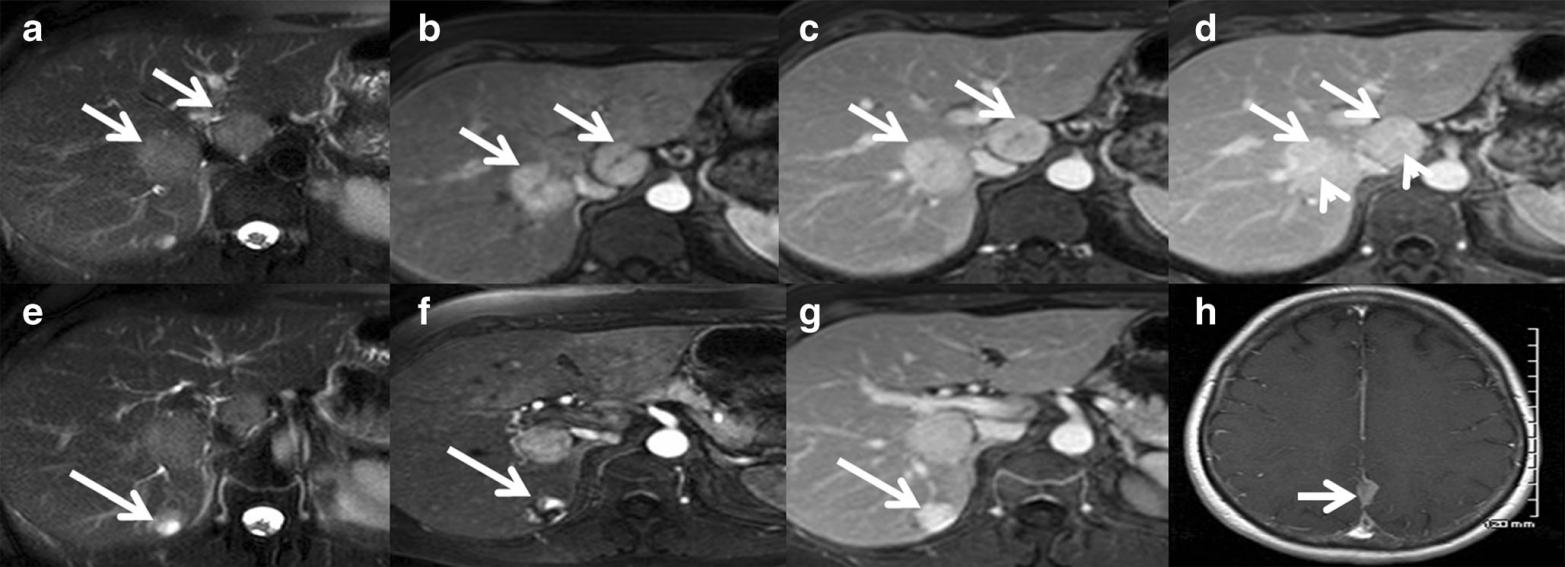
Multiple FNH syndrome in a 50-year-old female. (a) Axial T2W MRI shows two iso- to mildly hyperintense focal lesions (*arrows*) in segments 8 and 4 of the liver. Axial post-contrast MRI shows intense enhancement of the lesions (*arrow*) in the arterial phase (b) and persistent enhancement (*arrow*) in the portal venous (c) and delayed phases (d) with delayed enhancement of the central scar (arrowheads). (e) Axial T2W MRI in the same patient shows a small T2 hyperintense lesion in segment 7 (*arrow*). Axial post-contrast MRI shows peripheral nodular enhancement (*arrow*) in the arterial phase (f) with progressive centripetal filling (*arrow*) in delayed phase (g) in keeping with haemangioma. (h) Axial post-contrast MRI brain shows a small enhancing extra-axial lesion in the falx cerebri representing meningioma
